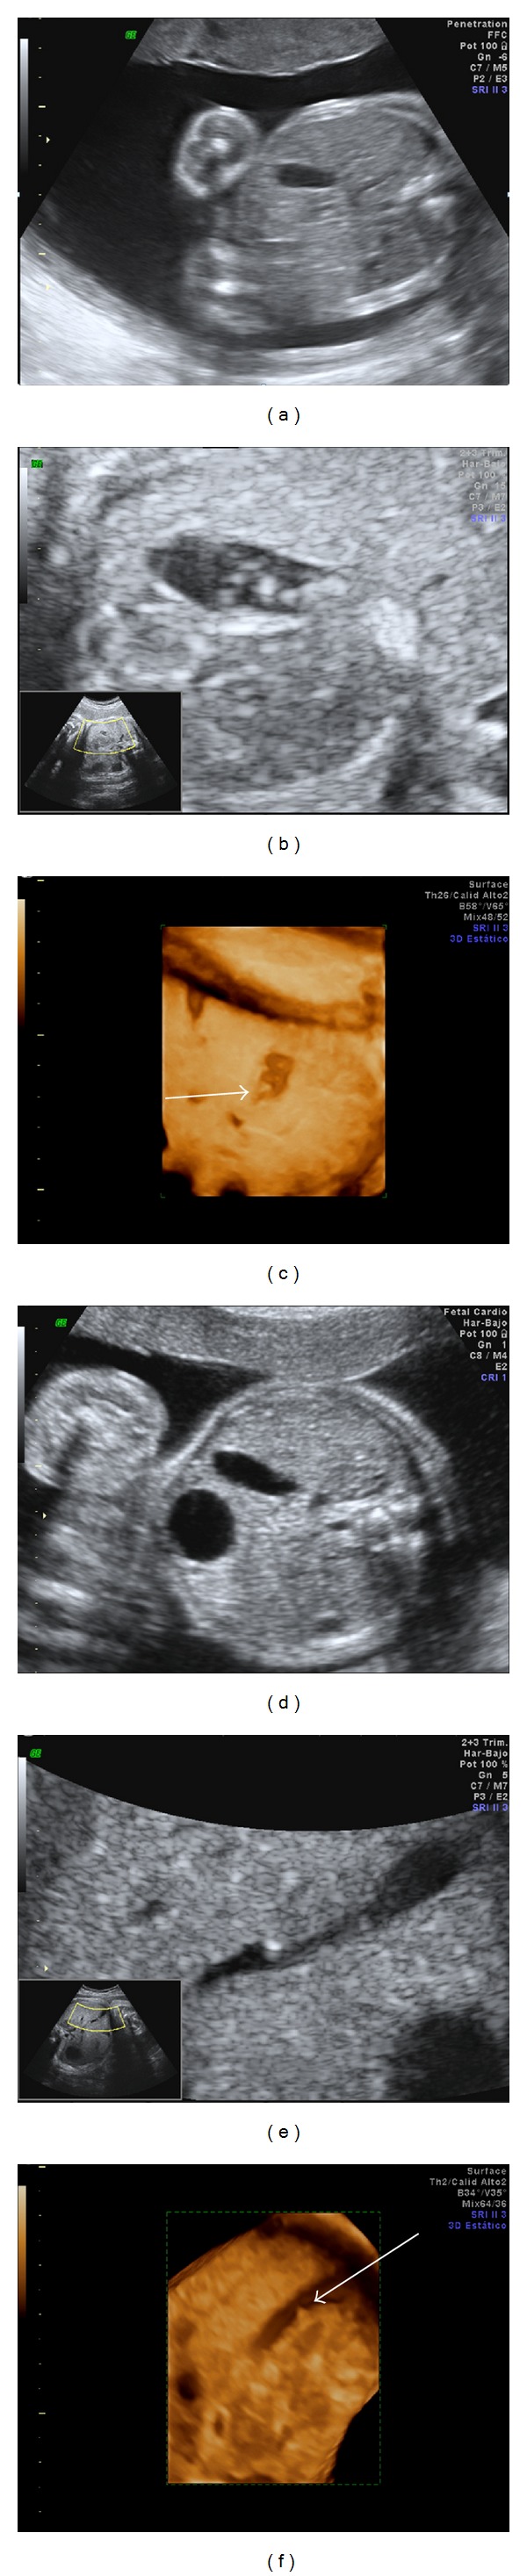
Case 1: ultrasound images of the distended gallbladder (a) containing biliary sludge (b) and 3D ultrasound of the same finding (arrow) (c). Case 2: ultrasound images of the distended gallbladder (d) showing biliary calculi (e) and 3D ultrasound of the same finding (arrow) (f).
